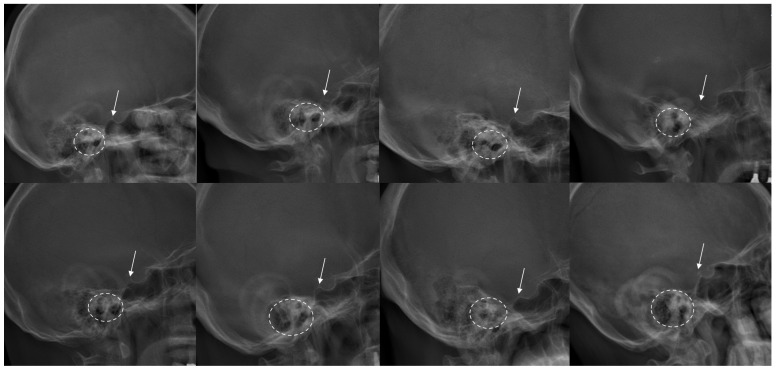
Various examples of patients’ head images in preoperative whole-spine X-rays. The posterior border of the sella turcica is indicated by a white arrow, while the external auditory meatus is indicated by a dotted circle. In most patients, the sella turcica appears clear and singular, while the external auditory meatus appears vague or duplex.
